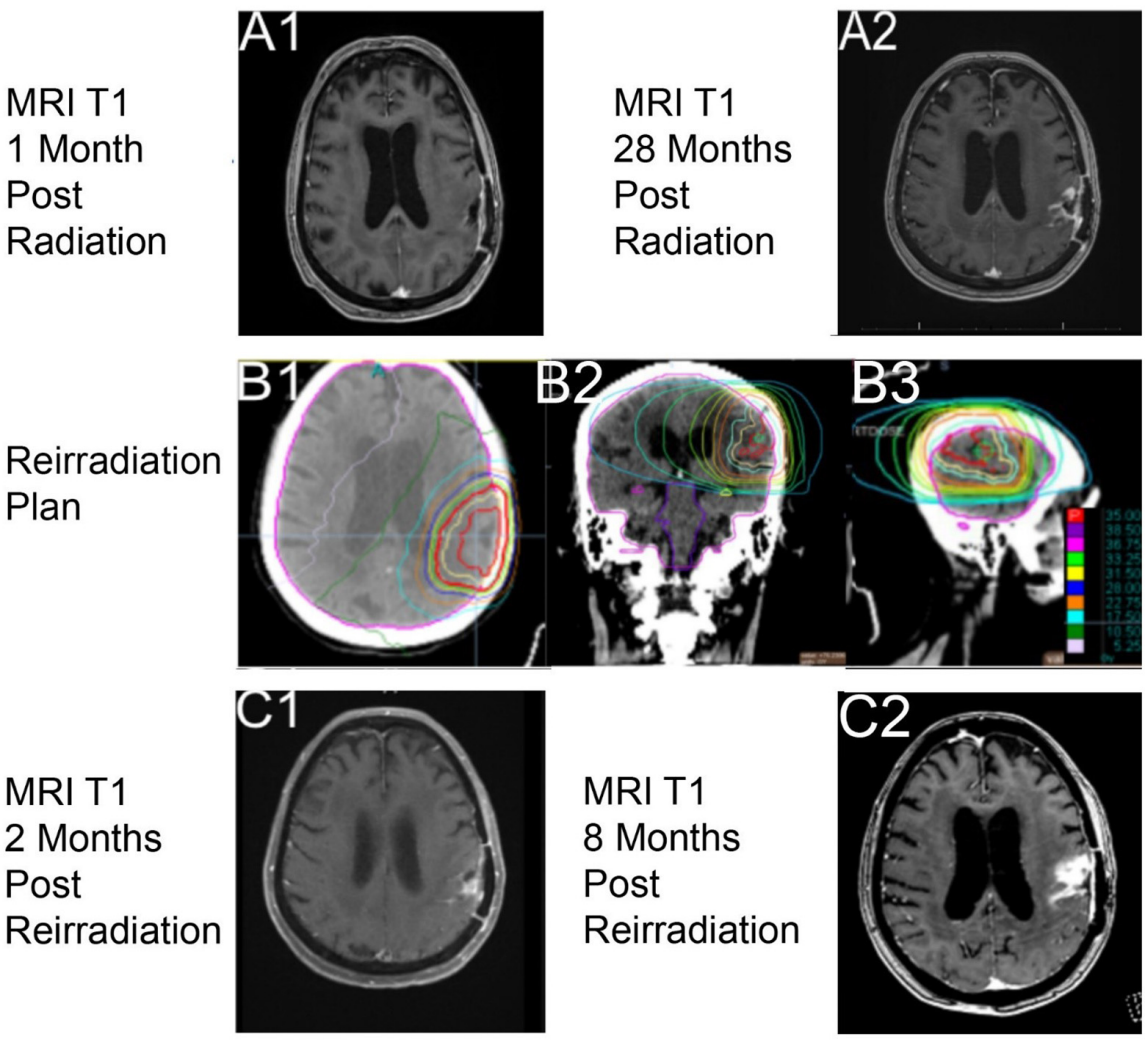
Imaging with T1 sequencing 1-month post-RT1, (A1) (axial through intraparietal sulcus), and 28 months post-radiation course 1, (A2) (axial through superior lateral ventricles). Reirradiation utilizing a noncontrast computed tomography (CT) with dose color outline and brain contoured as lavender, (B1–B3) (red is 35.00 Gy, purple is 38.50 Gy, pink 36.75 Gy, light green 33.25 Gy, yellow is 31.50 Gy, blue is 28.00 Gy, orange is 22.75 Gy, light blue is 17.50 Gy, dark green is 10.50 Gy, and light pink is 5.25 Gy). Imaging with T1 sequencing 2 months post-reirradiation, (C1) (axial through superior lateral ventricles). Imaging with T1 sequencing 8 months post-RT2, (C2) (axial through intraparietal sulcus).
